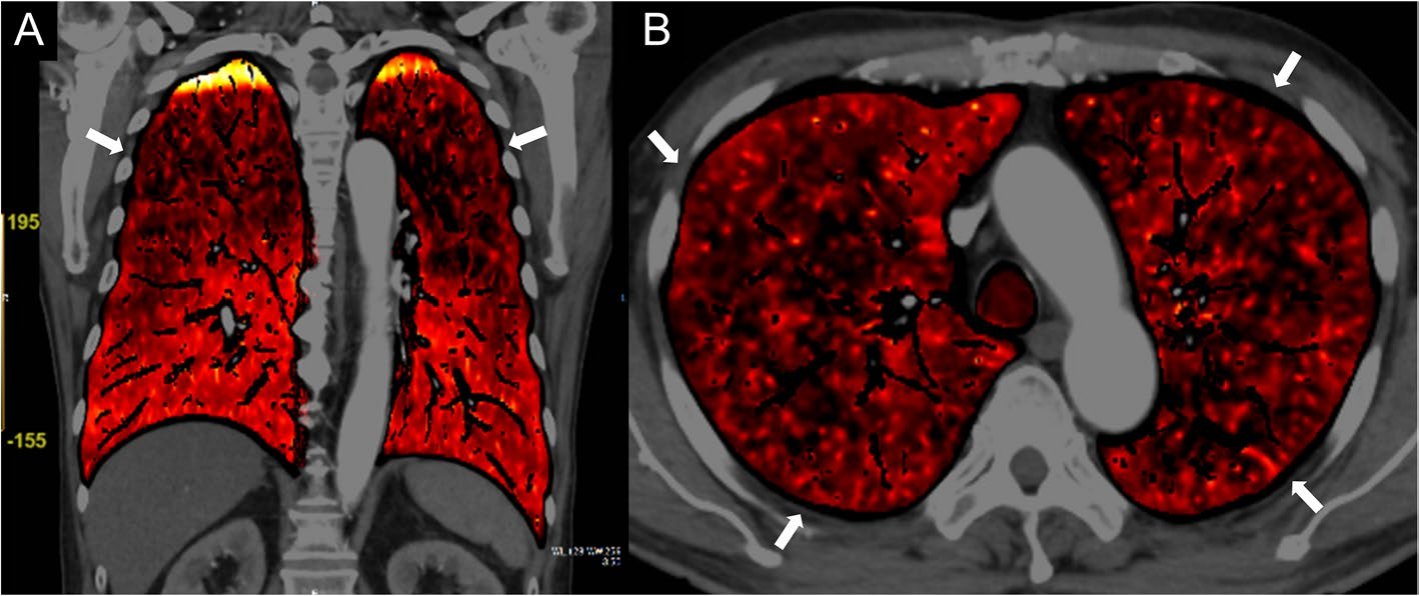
Dual-energy computed tomography images. A, B A color-coded iodine mapping image from the dual-energy CT shows decreased pulmonary perfusion in the upper lungs (arrows). The symmetrical, upper-lung dominant hypoperfusion was unusual for pulmonary thromboembolism and probably resulted from small vessel obstructive disease due to intravascular large B-cell lymphoma. The small yellow regions observed in the bilateral apices are attributable to hard beam artifact and do not imply high iodine concentration
